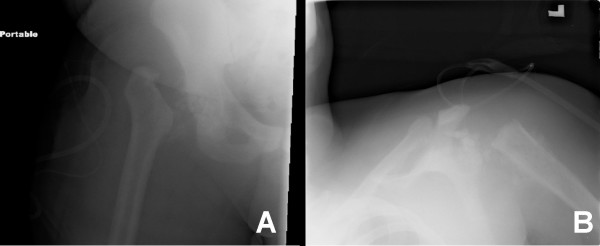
Radiographs of resection arthroplasties of hip and shoulder.
